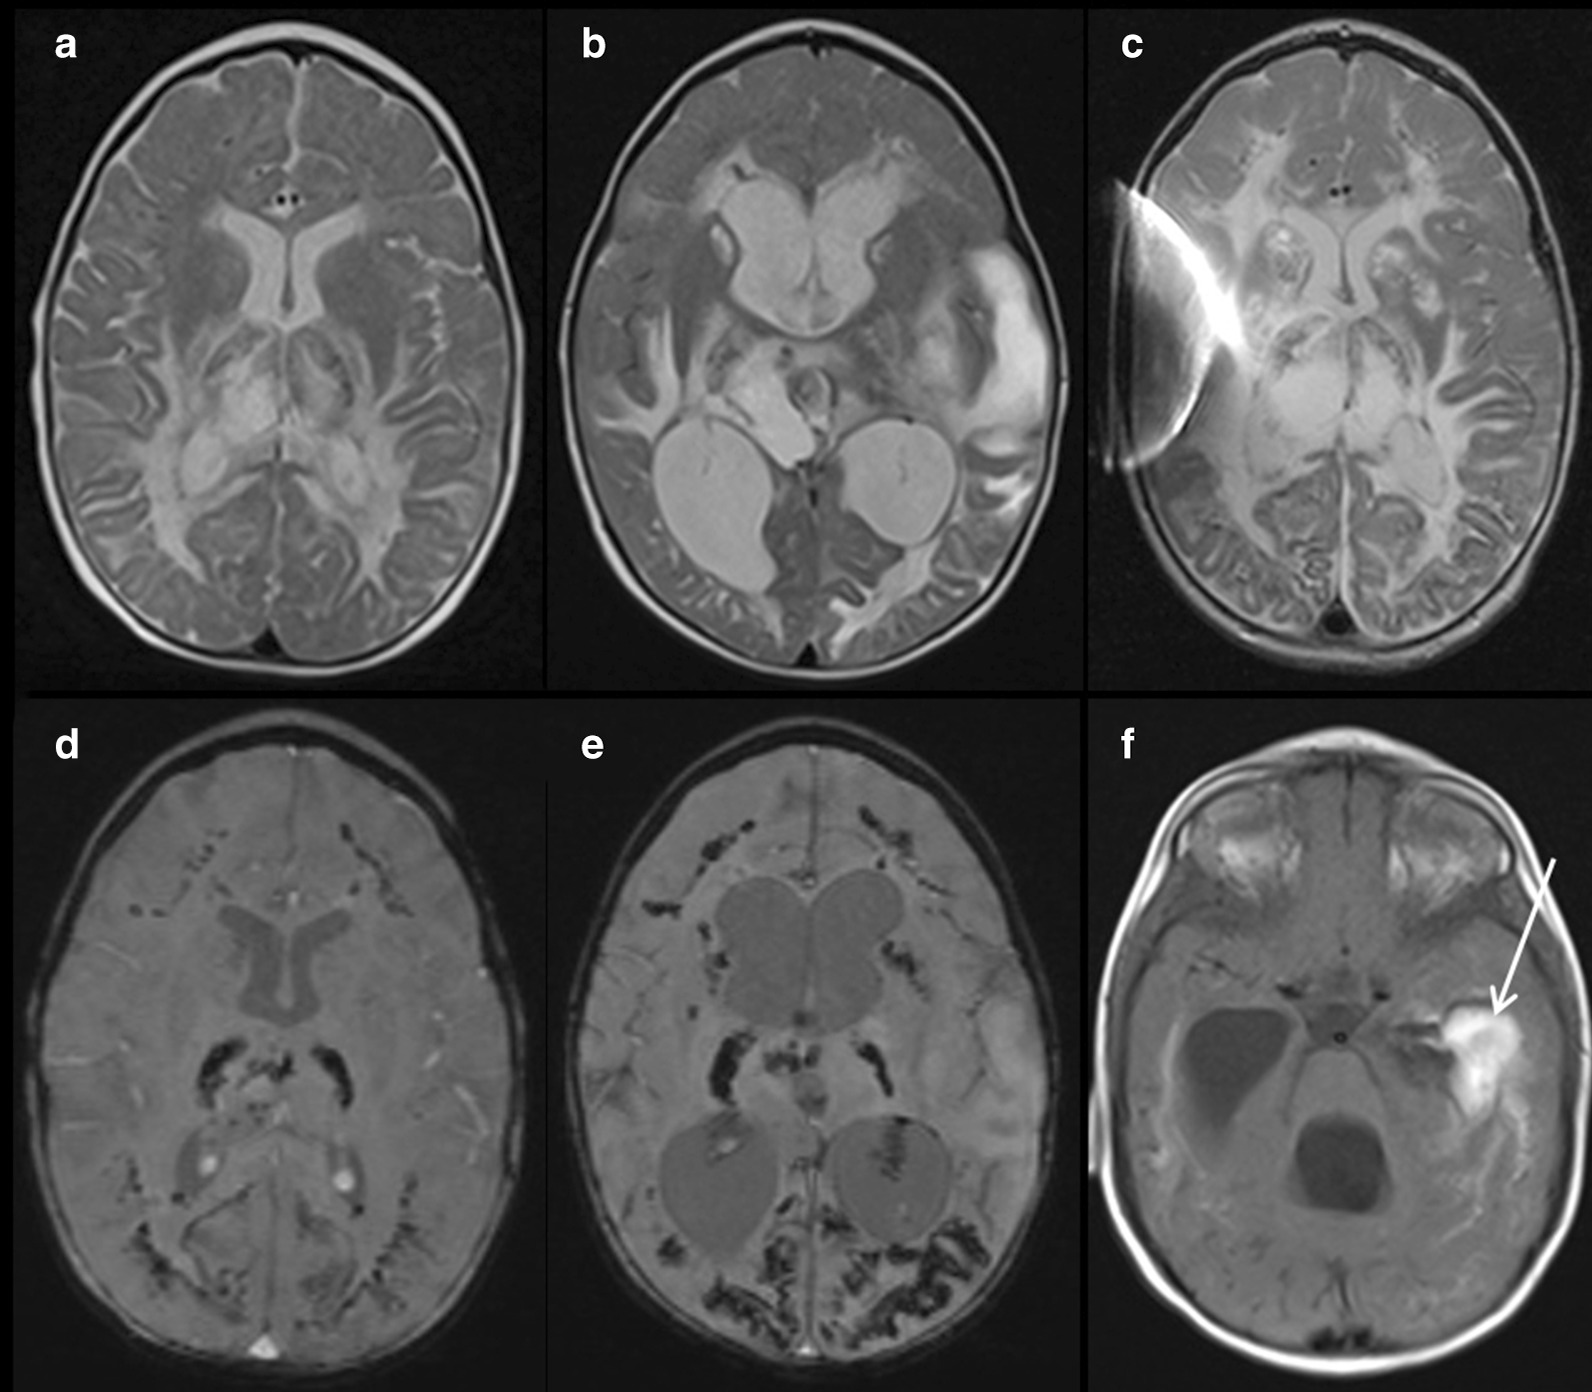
Axial T2-weighted images demonstrate progression of symmetric white matter lesions periventricular, within the thalamus, in the internal capsule, and the basal ganglia at the age of 1 year (a), 2 years (b), and 3.8 years (c). The corresponding axial susceptibility-weighted images (SWI) demonstrated progressive foci with susceptibility artefacts distributed symmetrically within the basal ganglia, the thalami, and on the border between gray and white matter (d, e), corresponding to calcifications. At the age of 2 years the patient suffered from a hemorrhage at the level of the left temporal horn with intraventricular breakthrough, shown as an area of high intensity (arrow) on the T1-weighted image (f), and hydrocephalic congestion (b, f), resulting in the need for ventricular-peritoneal shunt insertion. The artefact on the right side is due to the implanted shunt (c)
